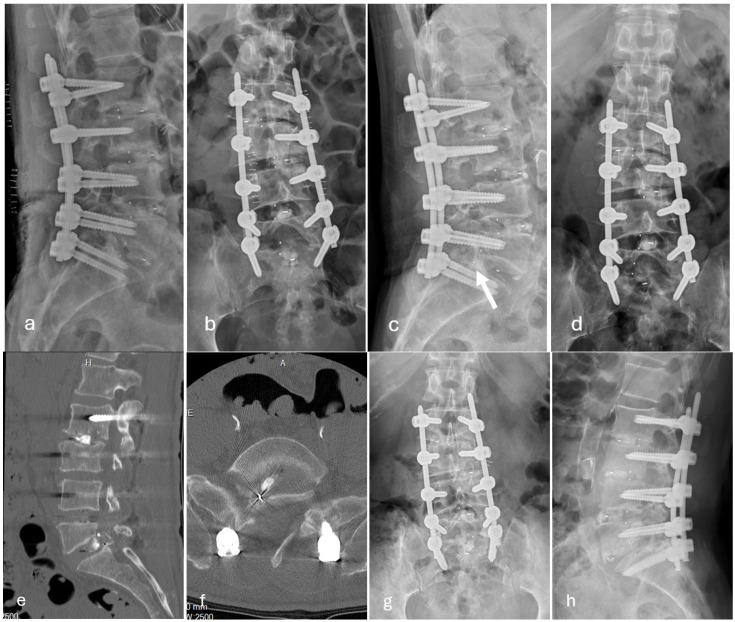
Representative imaging following minimally invasive transforaminal lumbar interbody fusion at L2/3, L3/4, L4/L5, and L5/S1. (a,b) Postoperative radiographs obtained on postoperative day 3 demonstrate appropriate interbody cage positioning at all operated levels. (c,d) Follow-up radiographs at 2 months after surgery show posterior migration of the interbody cage at the L5/S1 level (arrow). (e,f) Computed tomography images reveal posterior protrusion of the interbody cage into the spinal canal. (g,h) Post-revision radiographs demonstrate successful implantation of a new interbody cage via a contralateral left-sided approach.
